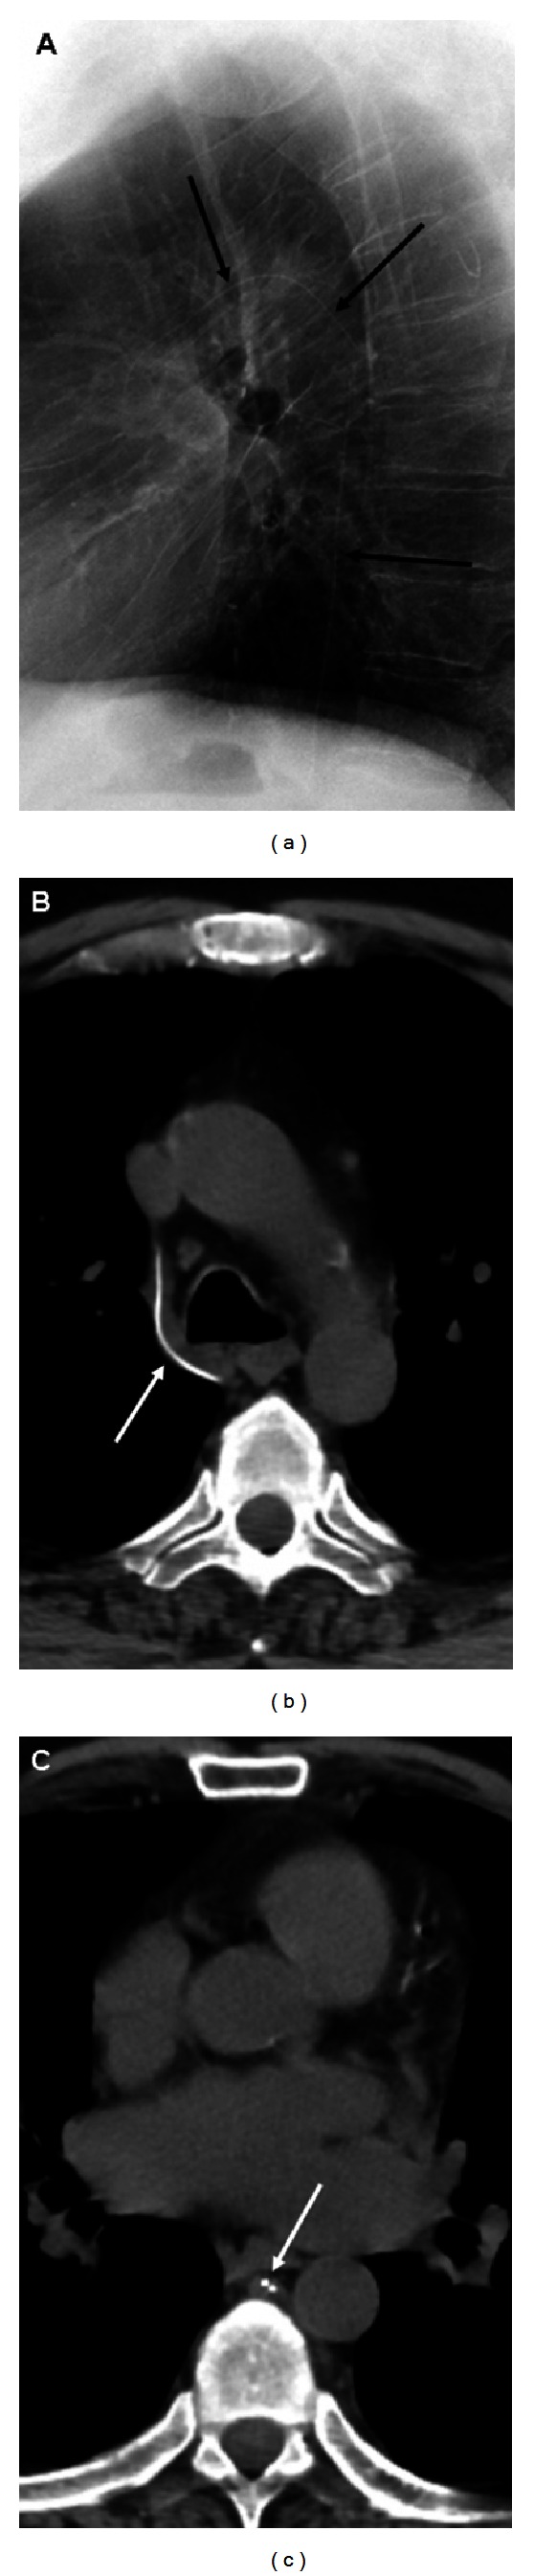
Lateral chest radiograph (a) and unenhanced axial chest CT images (b-c) show an opaque curvilinear density *arrows* within the azygos vein measuring approximately 29 cm in length. A short overlap *arrow *is present between the proximal and distal fragments (c).
